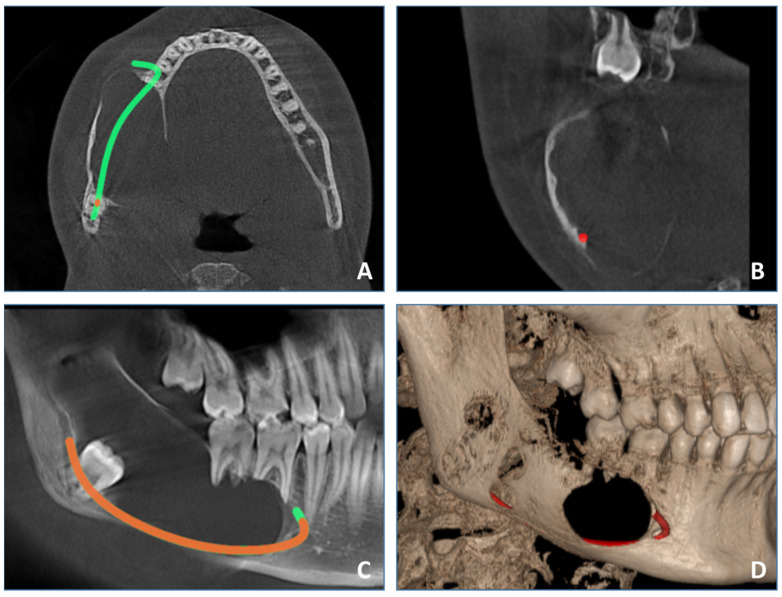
CBCT examination. (A)—axial view, (B)—coronal view, (C)—sagittal view, (D)—3D reconstruction.
